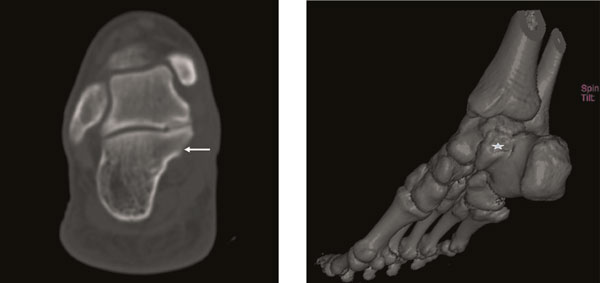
Axial CT scan (A) and three-dimensional (3D) imaging (B) taken at the 1-month of the second-operation of the foot shows no residual or recurrent lesion (arrow, star).
